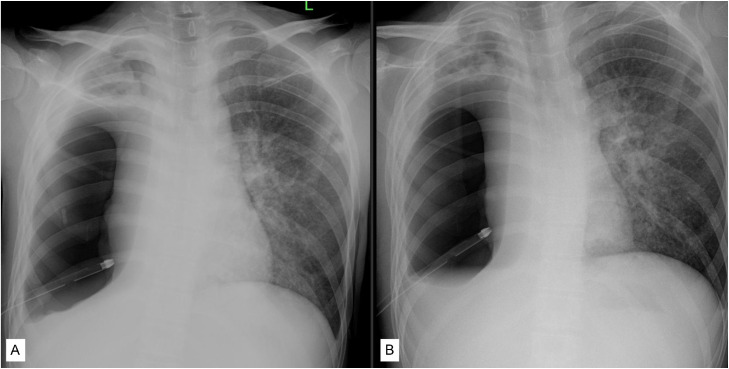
Chest radiography (A) initial chest radiograph at hospital admission showing right-sided pneumothorax, right pleural effusion, and left lung infiltrates; (B) after 7 days hospitalization, showed persistent right pneumothorax.
